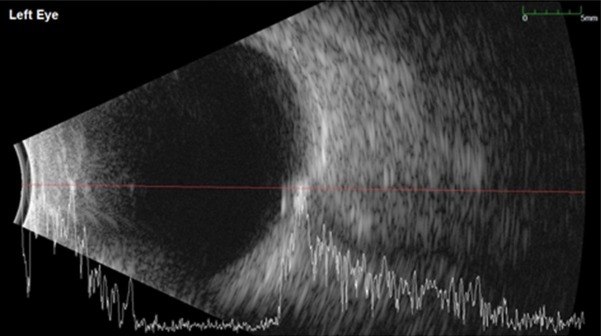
B-scan ultrasonography in the left eye
